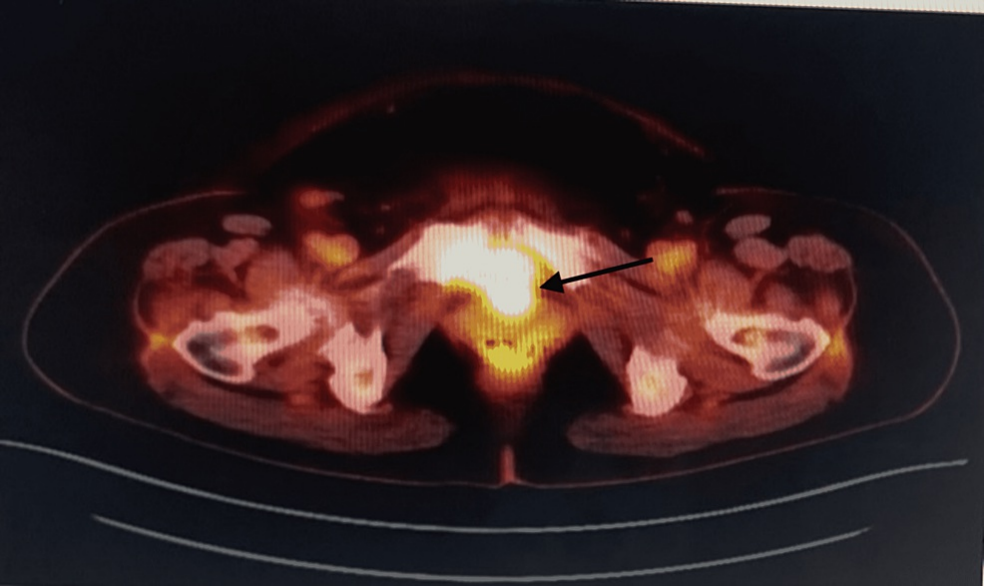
Initial staging PET-CT scan showing a malignant-appearing mass in the left posterior cervix. PET-CT: positron emission tomography-computed tomography
